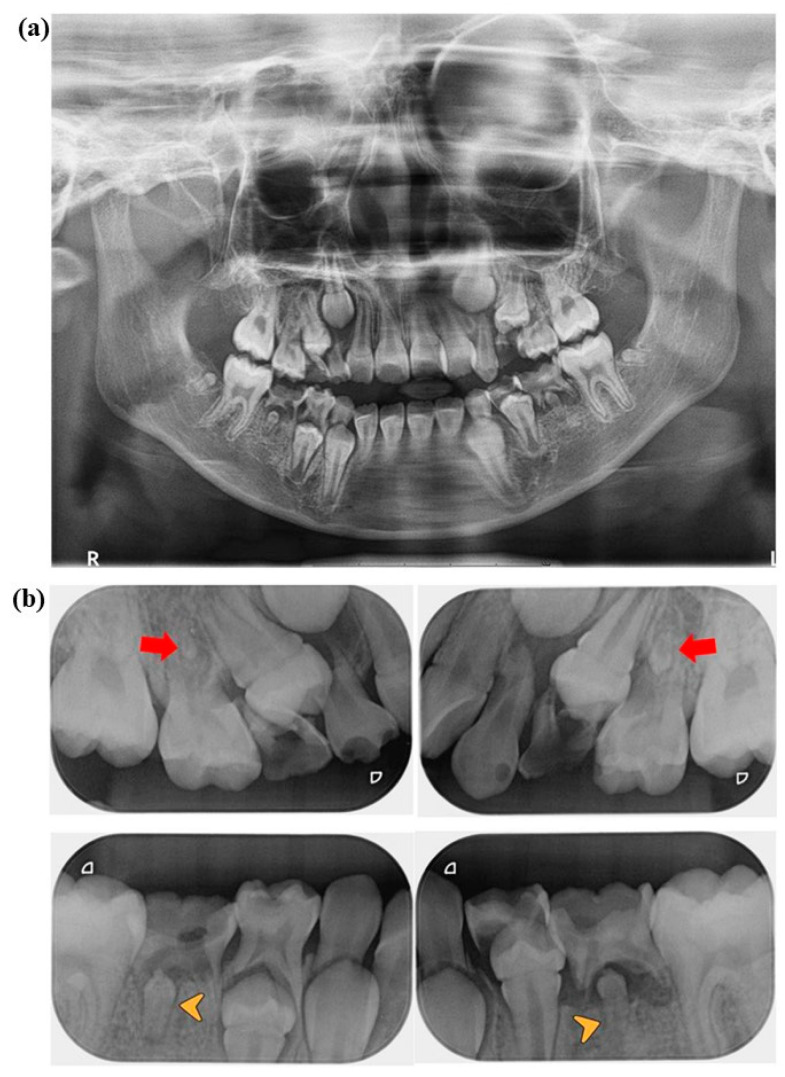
(a) Panoramic radiograph and (b) periapical radiographs were taken of the 7-year and 4-month-old patient (first visit). The film shows the patient’s primary teeth with a decayed-missing-filled-teeth index (DMFT) of 10. (b) The red arrows show rudimentary formations on tooth numbers 15 and 25. The yellow arrow heads show microdontia on tooth numbers 35 and 45.
